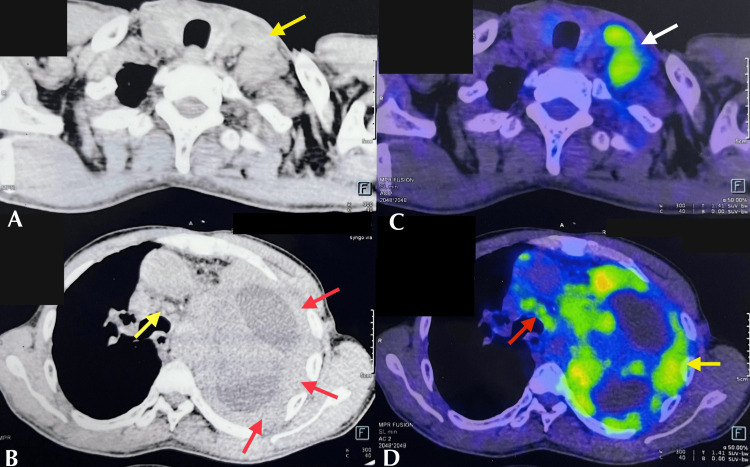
CECT and PET scan. (A) CECT showing few enlarged lymph nodes. (B) CECT showing nodular pleural thickening (red arrows) and few enlarged lymph nodes in the pre-tracheal region. (C) PET showing uptake in the left supraclavicular lymph node. (D) PET showing uptake along the pleural surface (yellow arrow) and multiple mediastinal lymph nodes (red arrow). CECT: contrast-enhanced computed tomography; PET: positron emission tomography
